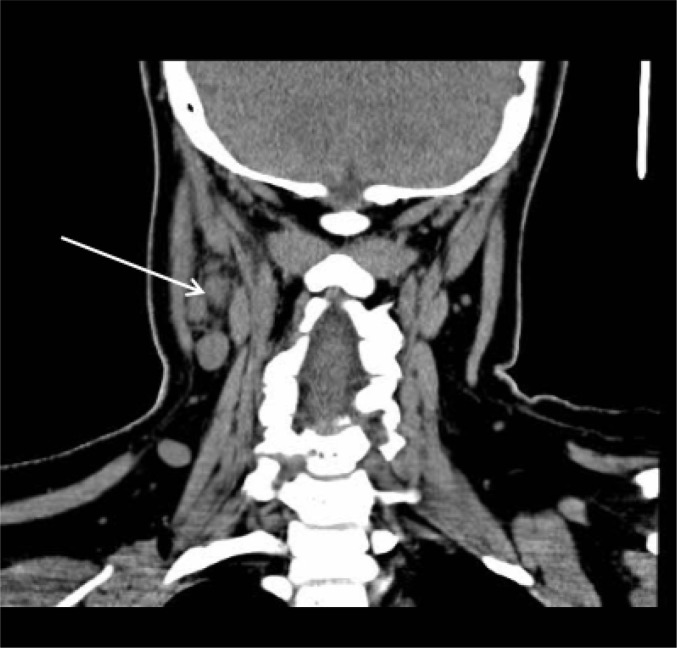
Computed tomography (CT) scan of the neck showing lymphadenopathy
